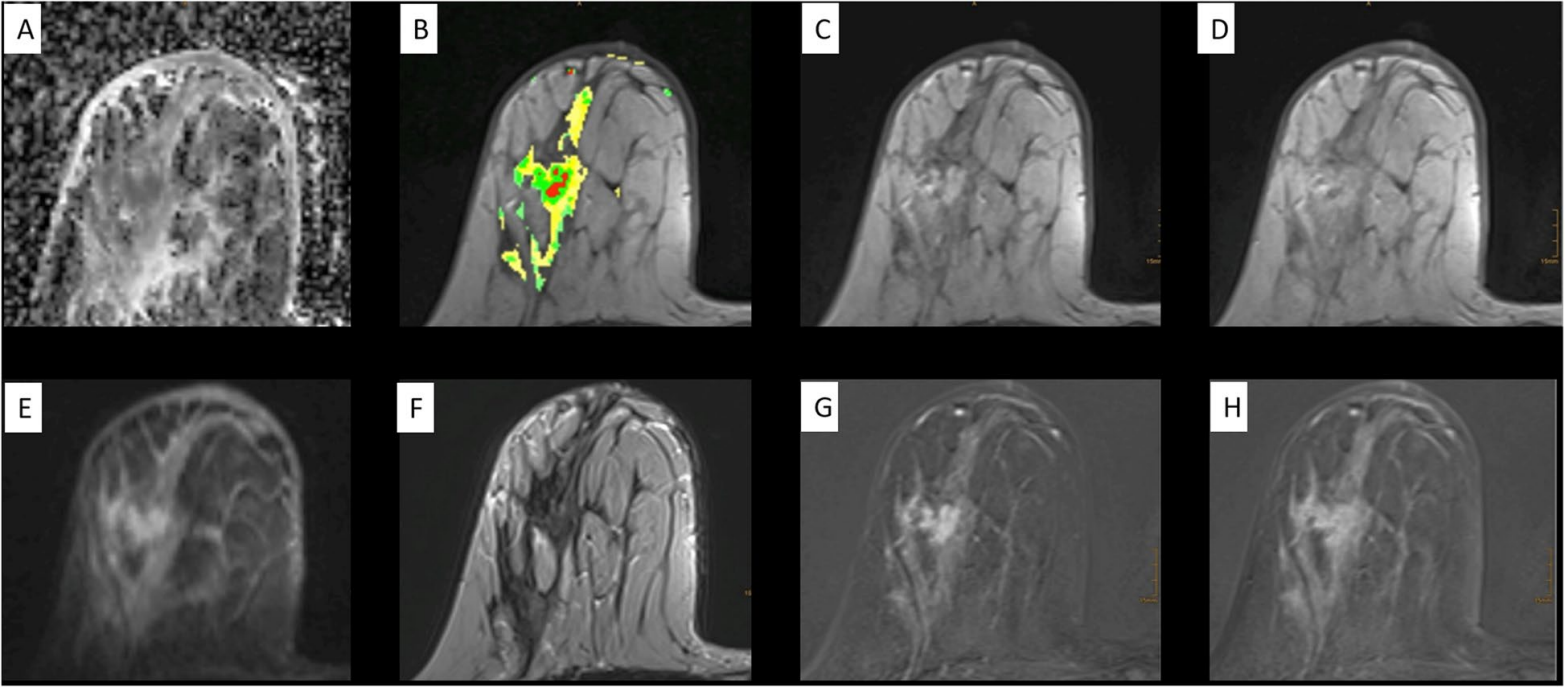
Standardised reading setup for comprehensive diagnostic and predictive/prognostic breast magnetic resonance imaging (MRI) at one-stop shop. A female patient with suspicious amorphous segmental calcifications on the right breast at mammography, breast imaging reporting and data system (BI-RADS) 4 diagnostic category (not shown). MRI was performed also for preoperative staging due to suspicion of extended ductal carcinoma *in situ*. Diagnostic MRI shows an extensive heterogeneous segmental non-mass enhancement predominantly with plateau dynamic pattern. A noncircumscribed mass with washout and heterogeneous internal enhancement (BI-RADS 5 diagnostic category) is located centrally within the non-mass lesions. Relevant prognostic findings are here washout, skin thickening, invasion of the nipple, and diffuse ipsilateral oedema (see also Figs. 5 and 6). Semantic criteria correspond to the MRI phenotype of an aggressive invasive breast cancer. P2-MRI results were confirmed by postoperative pathological examination (invasive cancer NOS, G3, Ki-67+++, triple negative, node positive). Apparent diffusion coefficient map (A); unenhanced T1-weighted gradient echo with colour overlap of the dynamic curve on a pixel-by-pixel basis (green/yellow/red = persistent/plateau/washout) (B); first (C) and last (D) contrast-enhanced T1 GRE; diffusion-weighted imaging obtained with *b* = 800 s/mm^2^ (E); T2-weighted turbo spin-echo (F); first (G) and last (H) contrast-enhanced subtractions. Diffusion-weighted imaging findings are highlighted in Fig. 7
